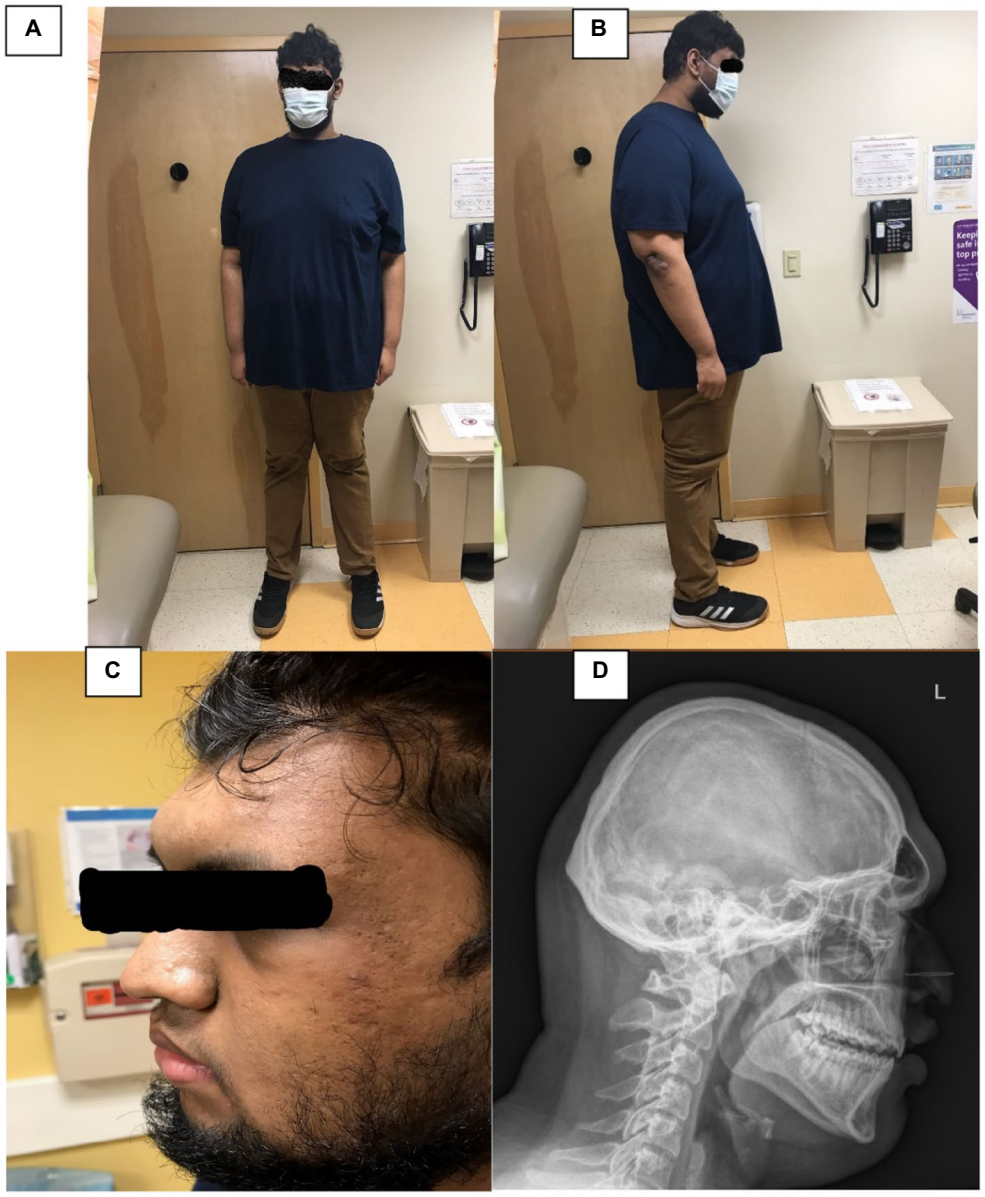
(A,B) Depict the patient’s frontal and profile views. (C) Depicts a closer view of his face, and (D) is a lateraral radiograph of his skull and neck.
